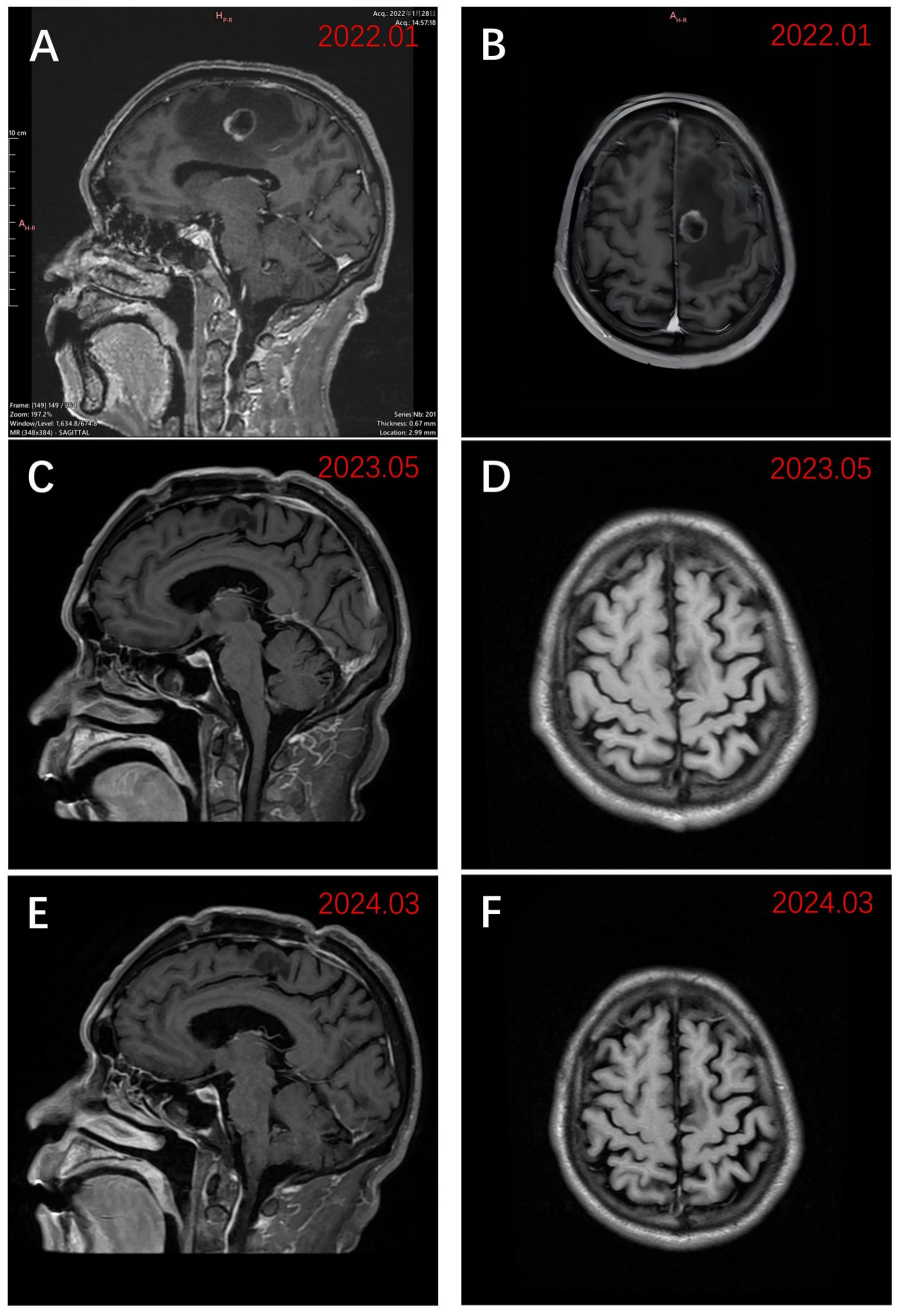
Sagittal and axial views of MRI images using T1WI enhancement. (A) Preoperative sagittal view in late January 2022 demonstrated a 20 × 19 mm lesion of the left parietal lobe; (B) Preoperative axial view in late January 2022; (C) Sagittal view in early May 2023 after receiving zimberelimab; (D) Axial view in early May 2023 after receiving zimberelimab. (E) The most recent sagittal view (March 2024); (F) The most recent axial view (March 2024).
